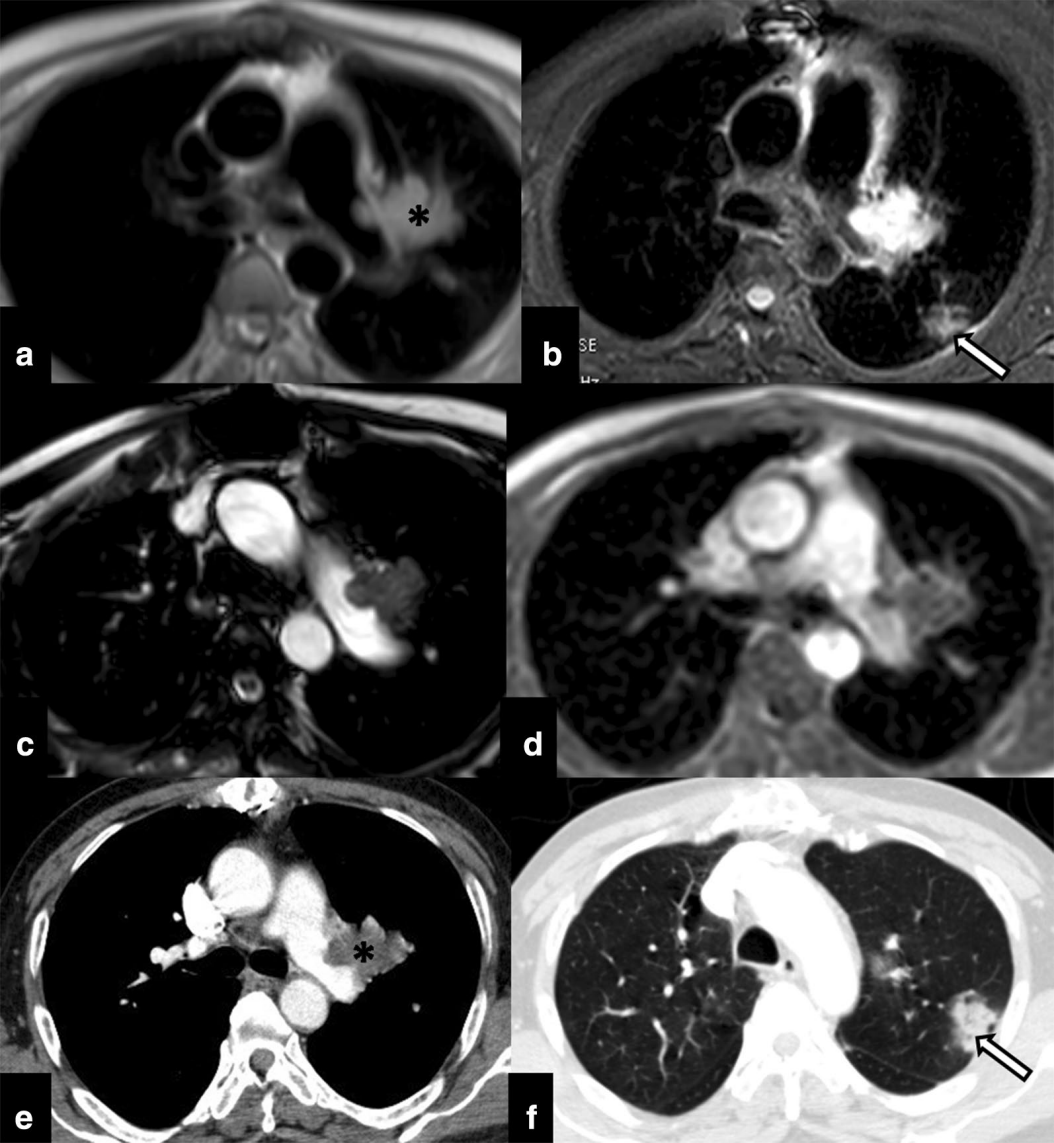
Post pulmonary endarterectomy cine-cardiac MRI (axial a, b, c, d T1W; b STIR, T2 and Post-contrast respectively) shows residual disease (*asterisk*) in the left pulmonary trunk with exophytic extravascular component. Focal patchy consolidation like opacities (*arrow*) were also seen in the lung parenchyma which were in favour of chronic thromboembolic phenomenon related infarcts presenting as consolidation (confirmed on post-contrast CT thorax: e mediastinal window and f lung window)
